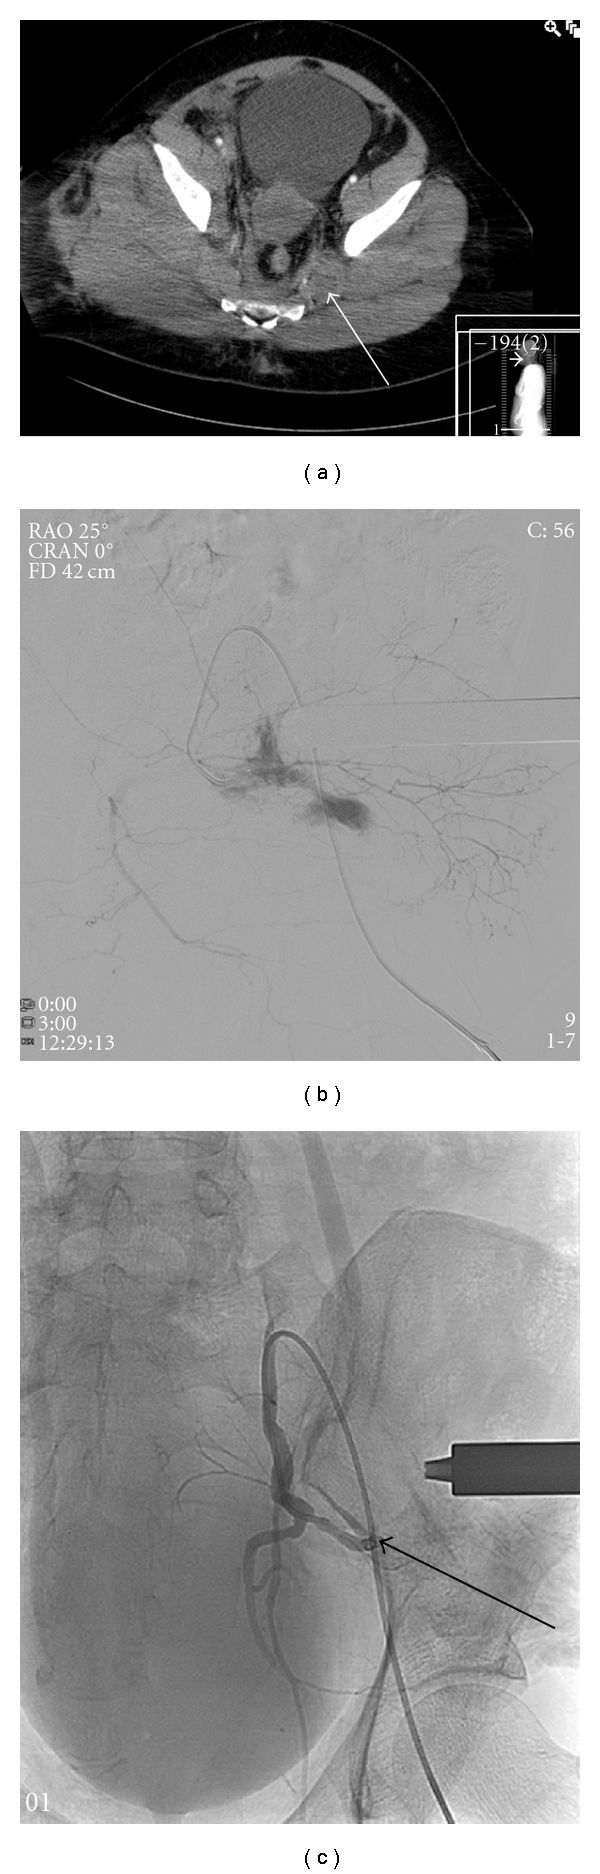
(a) Contrast-enhanced CT scan of a patient with a type C pelvic fracture after trauma. The arrow indicating a hematoma. (b) Selective catheterization of a branch from the left internal iliac artery showing a contrast blush, indicating an active bleeding. (c) Catheterization of the left internal iliac artery after coil embolization of the actively bleeding branch, with absence of any contrast blush (arrow indicating the coil in situ).
